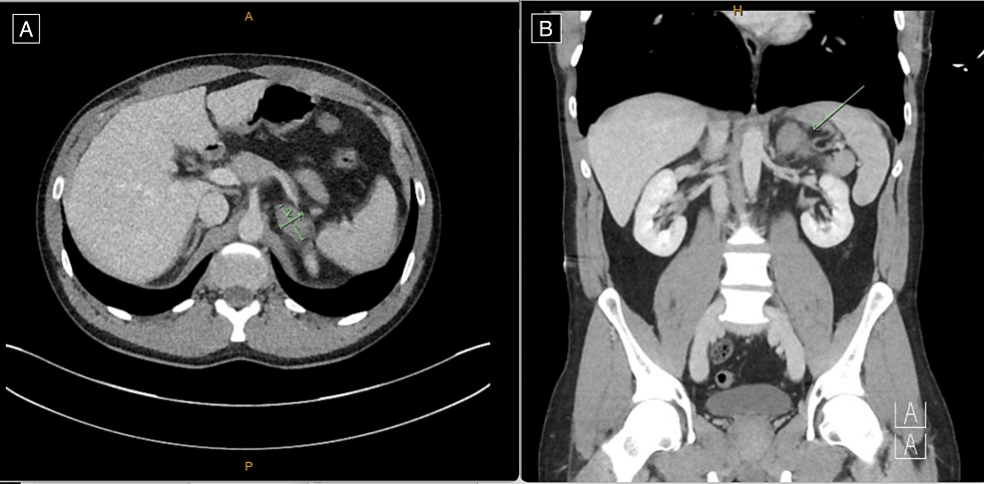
Computed tomography of the abdomen and pelvis demonstrating enlargement of the left adrenal gland with associated regional fat stranding and trace regional free fluid, in keeping with a traumatic adrenal gland haematoma, measuring 32 × 20 mm (A) on axial view and (B) on coronal view (see arrows).
